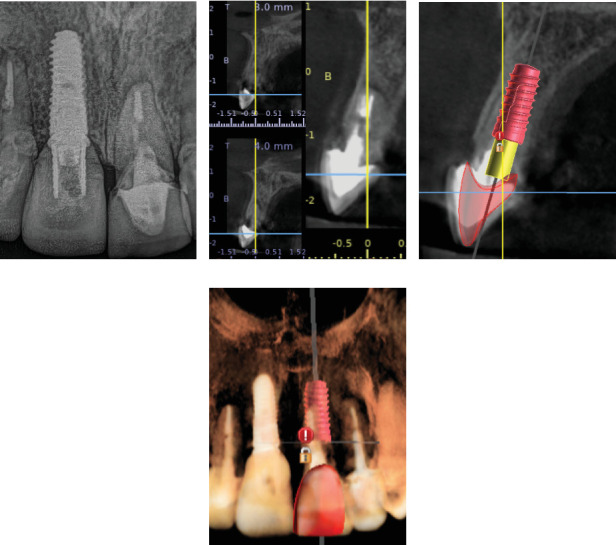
Initial imaging assessment. (a) Initial radiograph, (b) CBCT evaluation, (c) implant planning sectional view, and (d) implant planning frontal view.
